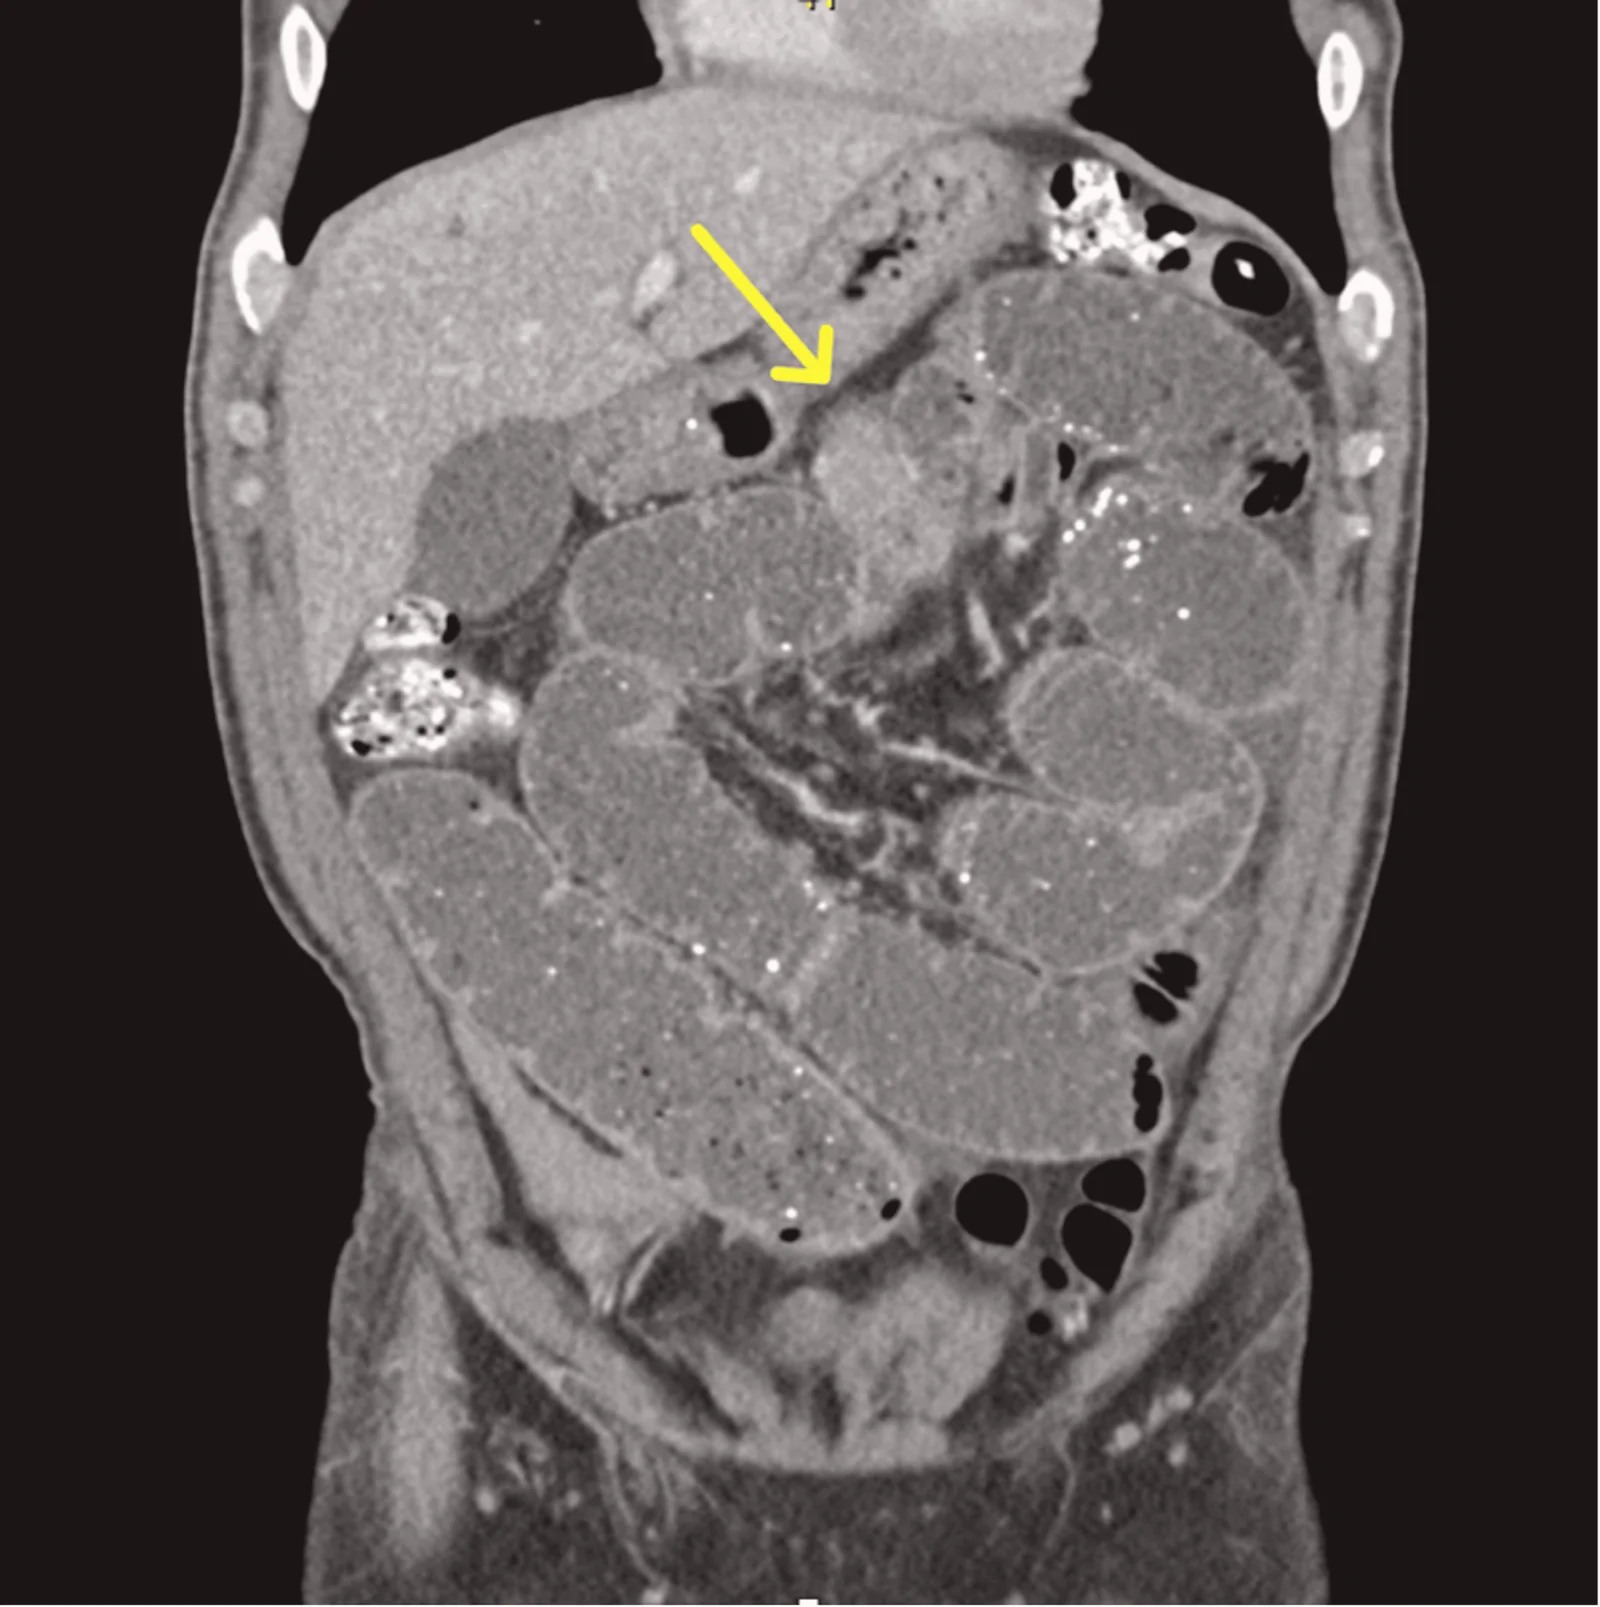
Coronal CT abdomen and pelvis demonstrating a soft tissue mass at the transition point (yellow arrow), leading to small bowel obstruction.
